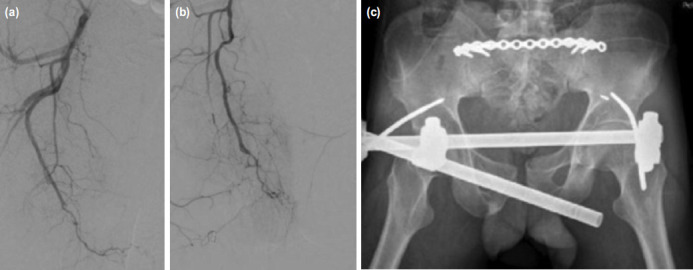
((a) Digital substraction angiography, selective right internal iliac artery (b) and superselective internal pudendal artery angiogram did not reveal any active contrast leak. (c) Post-operative radiograph of pelvis reveals posterior plating of the sacral fracture alongwith supra-acetabular fixator application for the pubic diastasis injury.
